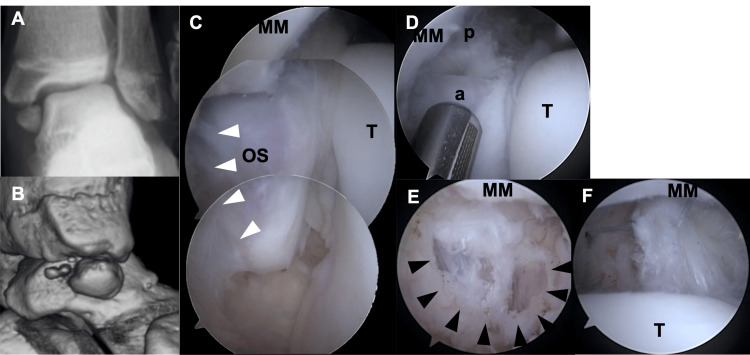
Preoperative images and arthroscopic findings (A) Valgus stress radiograph demonstrates widening of the gap between the medial malleolus (MM) and the ossicle (OS) and tilting of the talus. (B) Three-dimensional CT shows almost the whole anterior and posterior colliculus of the MM is defective and the OS corresponds to this defective part. The OS is composed of two separate bony parts. (C) The superficial component of the deltoid ligament (white arrowhead) is attached to the OS. The OS is clearly separated from the MM. (D) The OS is composed of an anterior large part (a) and a posterior small part (p). (E) The proximal end of the superficial component of the deltoid ligament is detached from the anterior large OS. (F) The proximal end of the deep posterior tibiotalar ligament detached from the posterior small OS is captured by repair suture wire. T: talus
